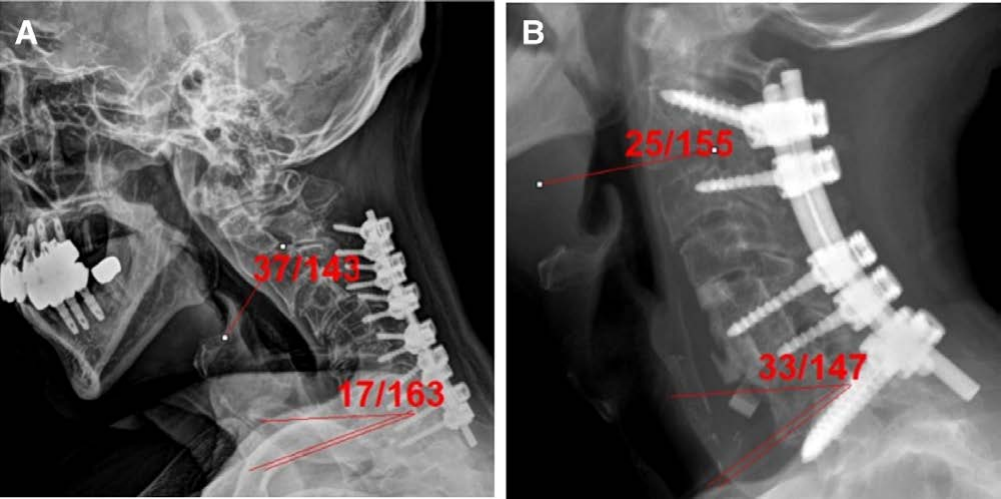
A 64-yr-old male patient complained of neck pain and difficulty raising his head. (A) A simple lateral X-ray image showed pulled-out screws and global kyphosis. Anterior and posterior approaches were performed, as well as C7 pedicle subtraction osteotomy. (B) Postoperative 1-year X-ray lateral image showed a well-balanced spine and complete fusion.
